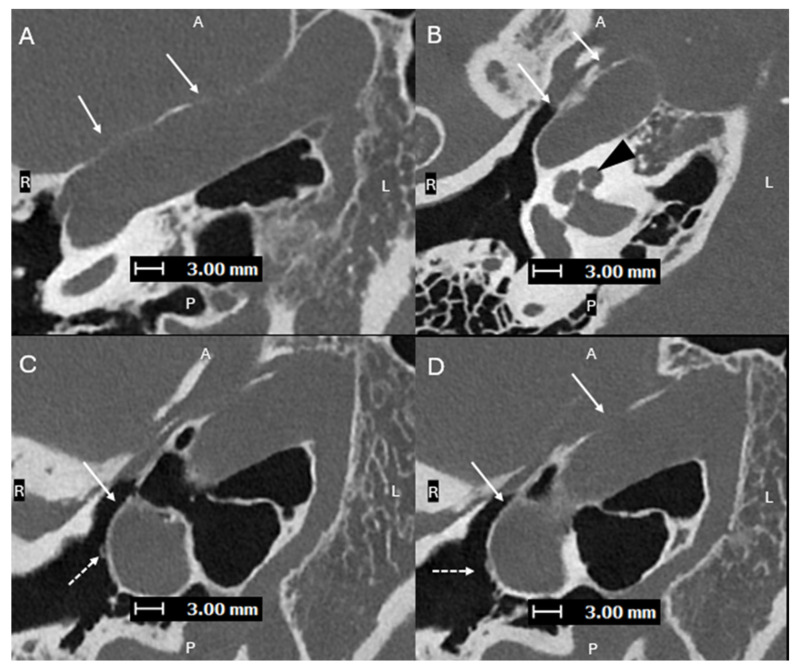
Axial oblique images (superior to inferior (A–D), with A, P, L, R for orientation donating anterior, posterior, left, and right respectively) from a PCD-CT temporal bone study—with same acquisition and reconstruction parameters as Figure 1—demonstrating a lateralized R ICA (solid arrows). (B) shows the ICA lateral to the midportion of the basal turn of the cochlea (black arrowhead). (C,D) show the normal R ITC visualized laterally (dashed arrows), proving that this is a lateralized ICA and not an aberrant ICA. A lateralized ICA is defined as the genu of the vertical and horizontal segments of the petrous ICA positioned lateral to the midportion of the basal turn of the cochlea in the axial plane. Importantly, the ITC is not affected, which helps to distinguish a lateralized ICA from an aberrant ICA [8]. Skull base foramina, such as the inferior tympanic canaliculus, are much better seen on PCD-CT, which can greatly help when trying to differentiate between an aberrant ICA and a lateralized ICA, as shown in cases 2 and 3 [9]. On EID-CT, it can be difficult to differentiate between a lateralized ICA and an aberrant ICA.
